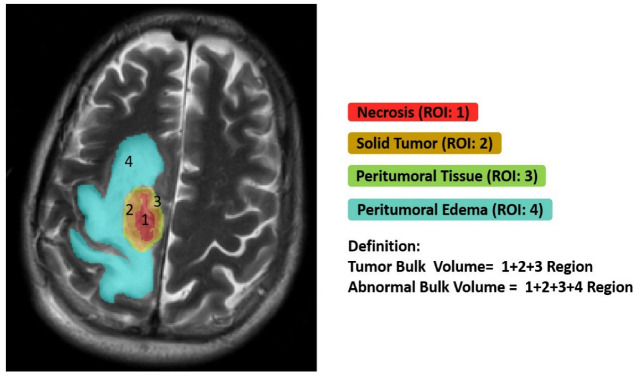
GBM derived from T1-CE MR images for individual measurement in specific necrosis, solid part tumor, peritumoral tissue, and edema regions of right-side frontal lobe volume in a subject. TBV represents the addition of these tumor features. The ABV is represented by hyperintensity extracted from T2-FLAIR images. Edema is the difference of tumor bulk from abnormal bulk volume.
